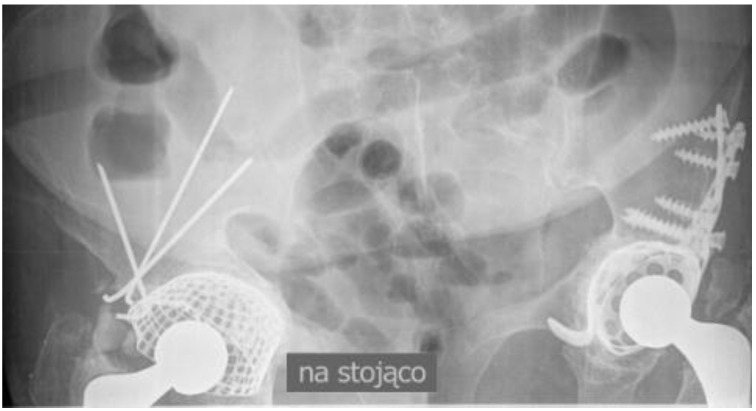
An X-ray of the abdomen visualizing both hip joints 13 years following successful right hip revision arthroplasty.
